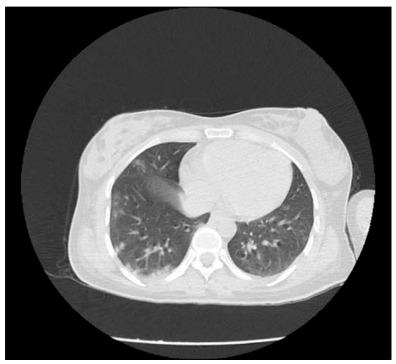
Thoracic computed tomography.
